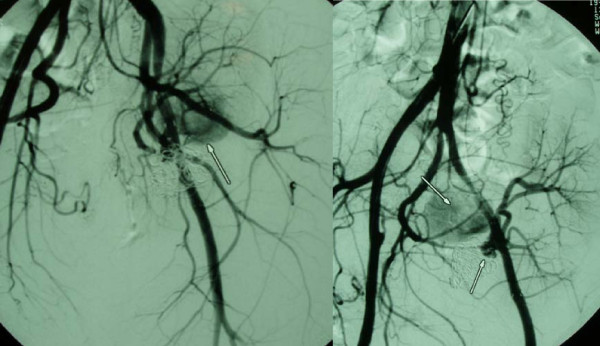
Clinical symptoms and pseudoaneurysm revealed by Color Doppler Ultrasonography reappeared some days later and was verified by angiography (arrows).
